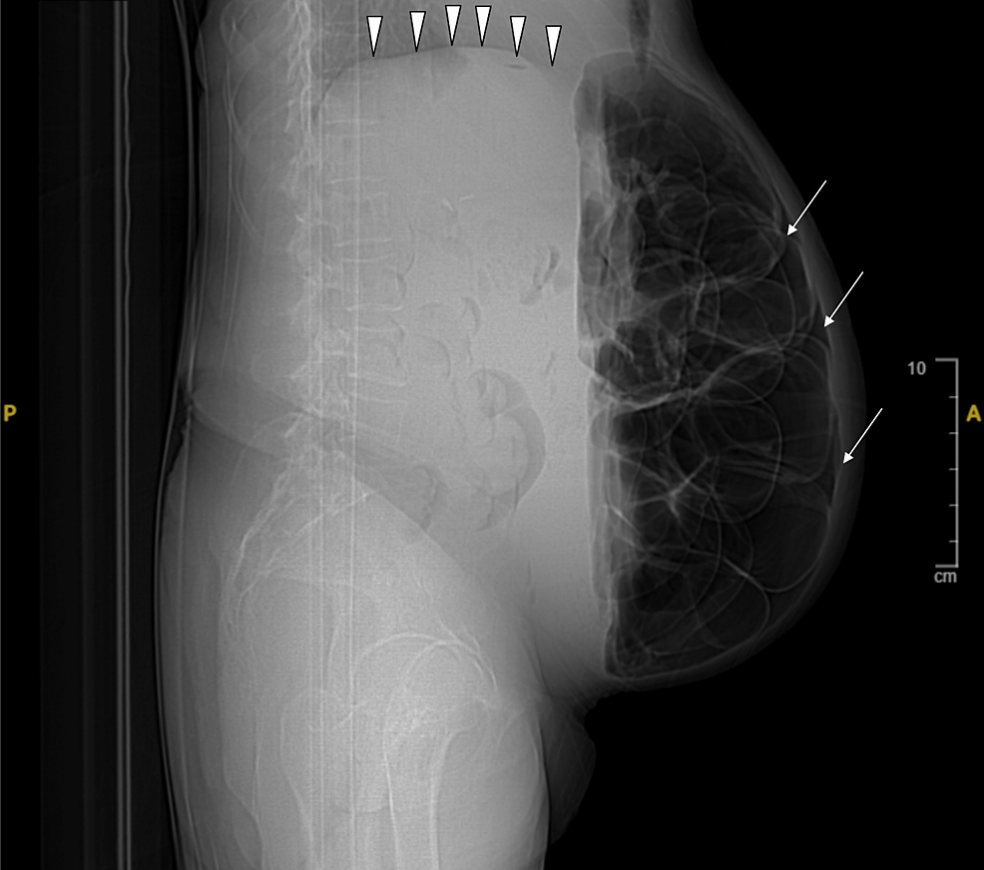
X-ray of the abdomen and pelvis without contrast, supine position, lateral view displaying diffuse ascites (white arrowheads) and pneumoperitoneum (white arrows).
